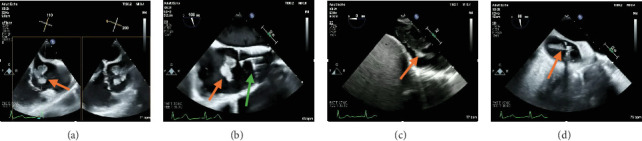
Intraprocedural transesophageal imaging displaying (a) recurrent right atrial thrombus extending from IVC (orange arrow). (b) AngioVac debulking (green arrow) of right atrial thrombus (orange arrow). (c) IVC thrombus extending from hepatic vein (orange arrow). (d) AngioVac thrombectomy of hepatic vein (orange arrow).
